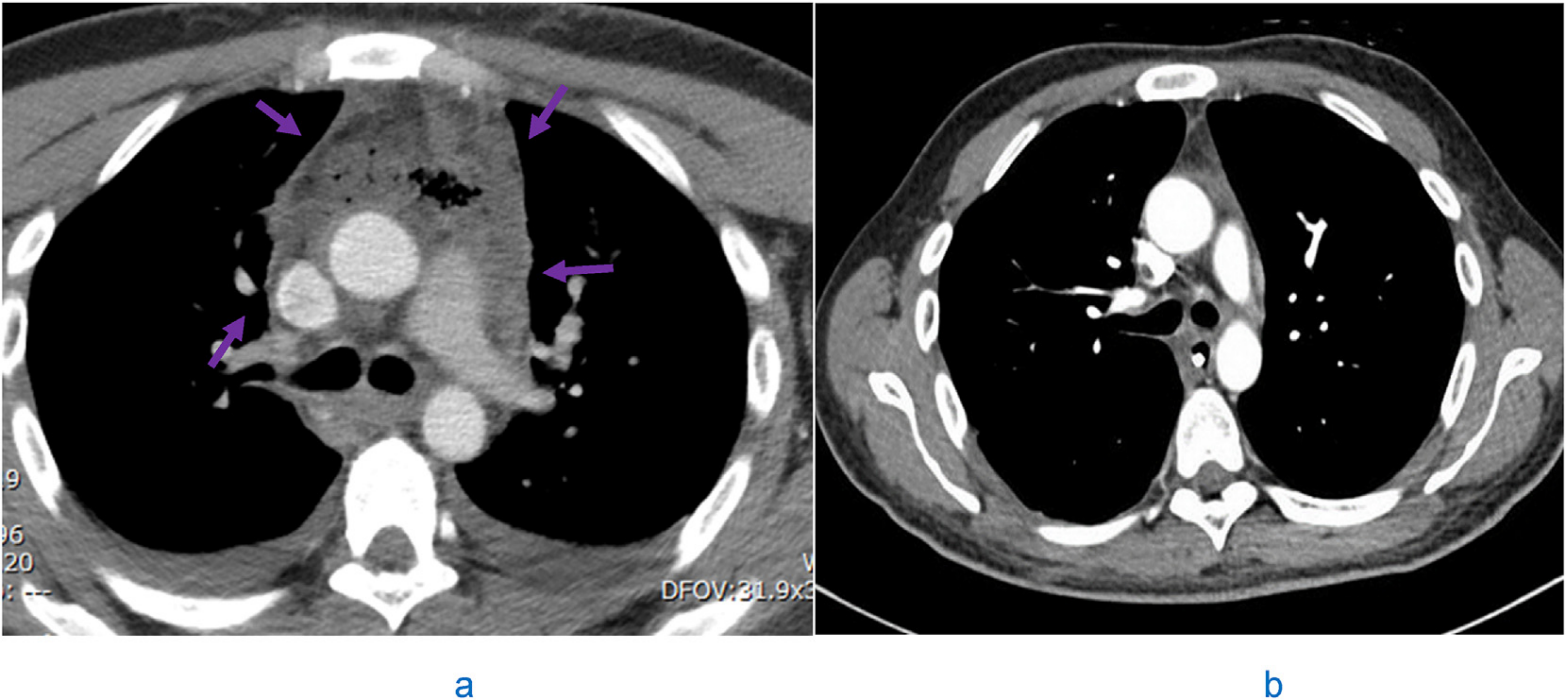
a: Axial section of contrast-enhanced computed tomography scan of the neck and thorax at the level of the tracheal bifurcation shows descending necrotizing mediastinitis complicated by abscess formation in the superior and anterior mediastinum (purple arrows). b: Axial section of contrast-enhanced computed tomography scan of the thorax shows total resolution of the mediastinal abscesses (performed three months post-operatively).
